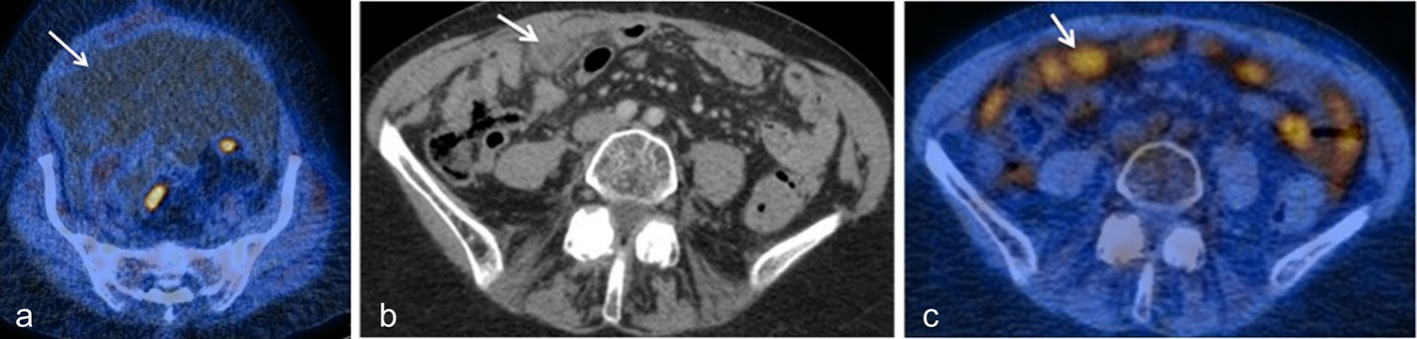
A 67-year-old female with endometriod carcinoma of the ovary. (a) The primary disease in the pelvis is poorly FDG avid (arrow). (b, c) A cystic deposit in the serosa, equally poorly FDG avid and therefore masked by bowel, better demonstrated on the CECT. CECT, contrast enhanced CT; FDG, fluoro-2-deoxy-D-glucose.
